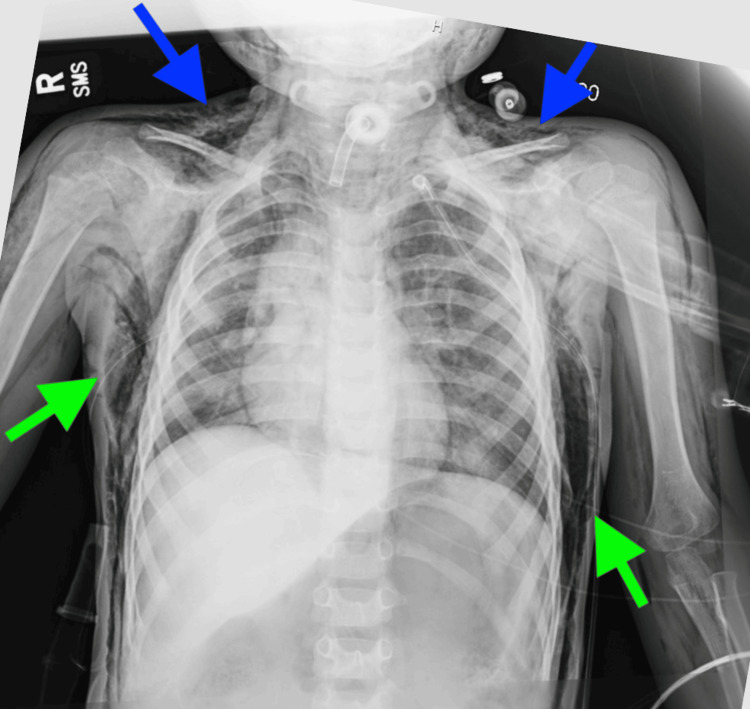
Chest radiograph findings Demonstrating bilateral chest tubes (green arrows) with re-expansion of both lungs. Residual subcutaneous emphysema persists (blue arrows).
